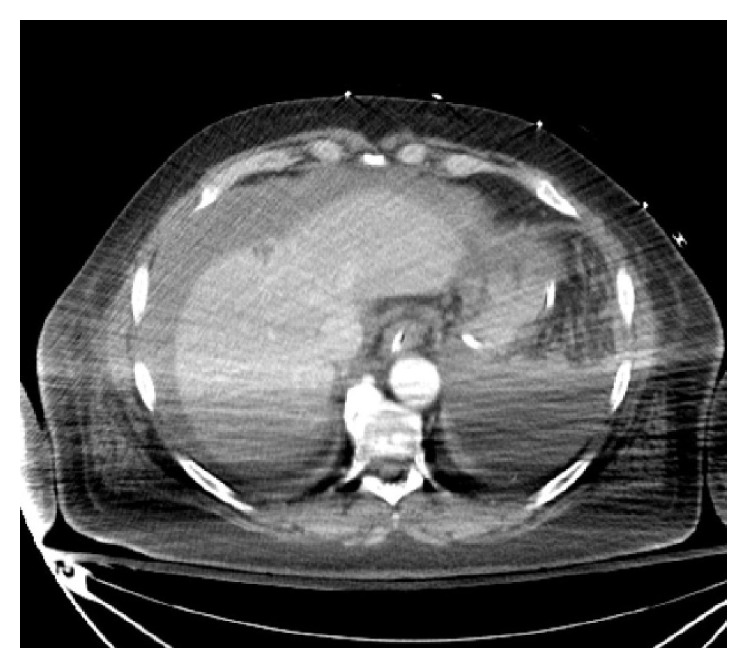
CT scan of abdomen and pelvic revealing hemoperitoneum and mass-like lesion in segment 4 of liver.
